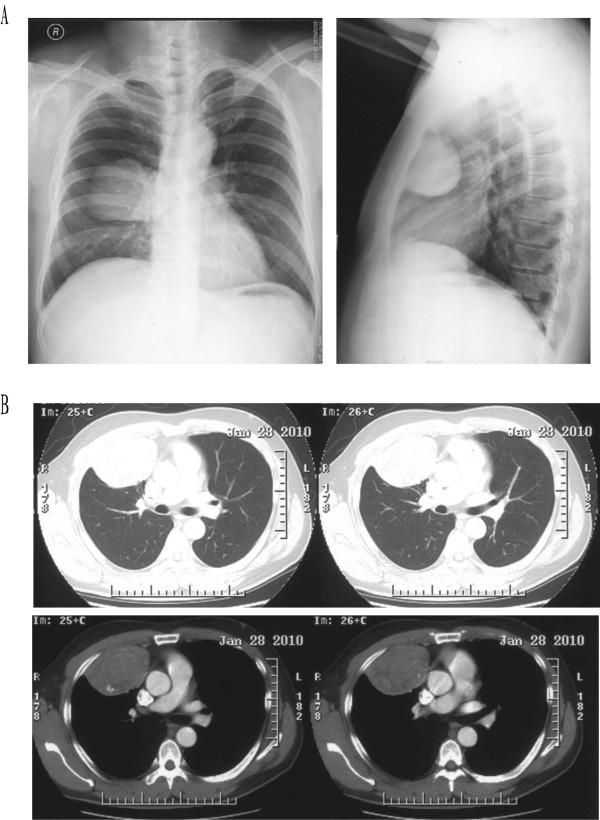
Chest images (A) Chest radiograph. A mass is seen in the right middle lung field on chest roentgenogram; (B) Chest contrast enhanced computed tomography scans. A solid, round pleural mass is seen in the right anterior chest wall, with heterogeneous density and calcification. It is well capsulated and compresses the lung parenchyma without any sign of infiltration of lung or chest wall.
